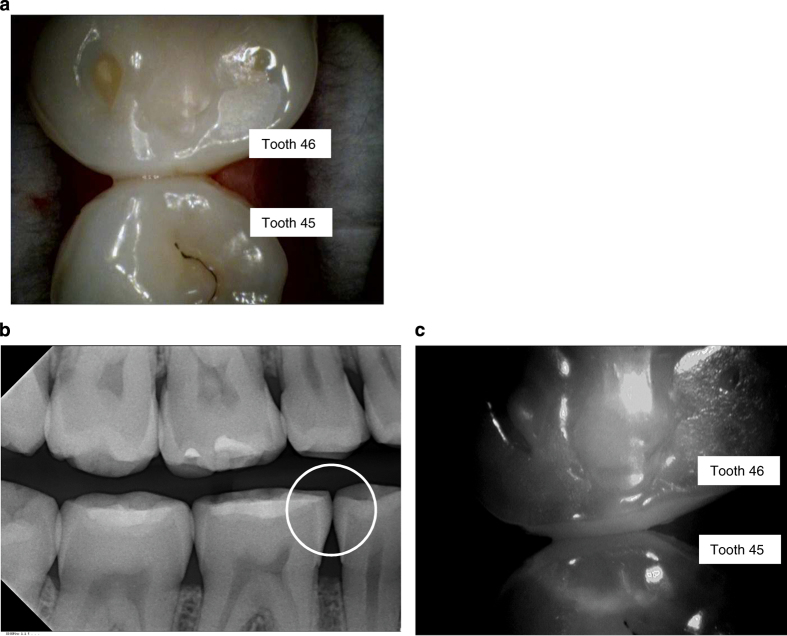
Examples of images using the VistaCam iX Proxi head. In some NIR images, a halo can be seen in the tooth, which is presumably due to the fact that light gets reflected from the round glass on the inner lens of the camera. The reflection can only be seen on white background. This lens has been replaced in the new commercially available device by a specifically coated lens that does now longer cause this irritation. (a) Image of teeth with the intraoral camera (45 distal, 46 mesial). (b) Corresponding bitewing radiograph (no radiolucency seen in 45 distal/46 mesial). (c) Corresponding NIR image.
